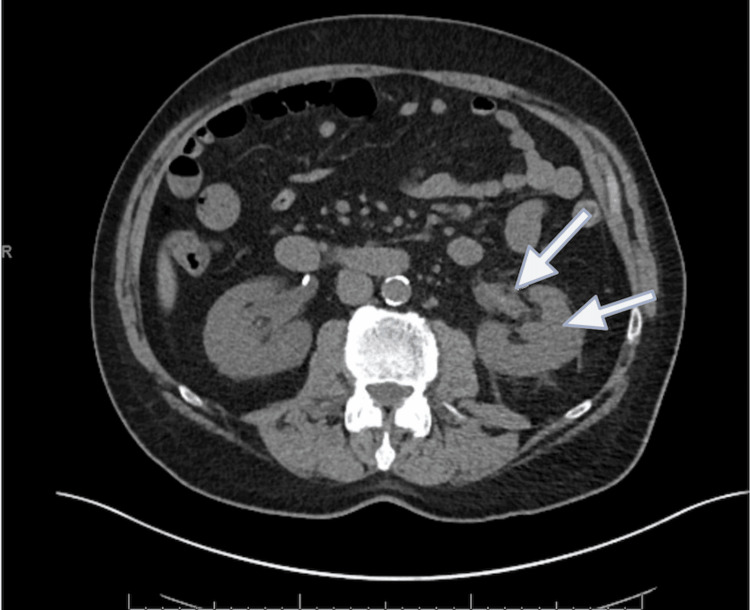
CT abdomen/pelvis on later presentation showing left renal hemorrhage and hydronephrosis (arrows)
